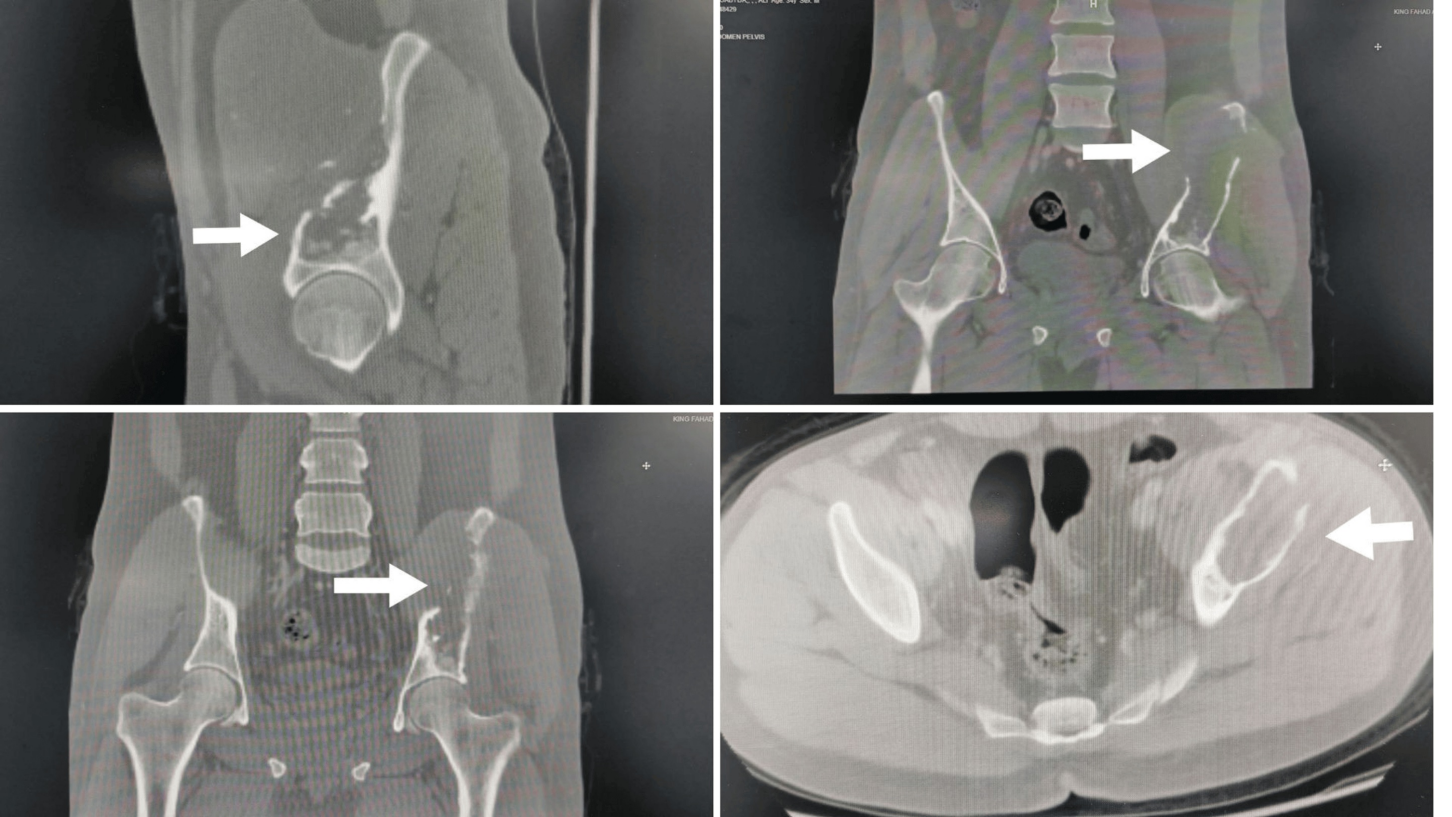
CT pelvis coronal, sagittal, and axial (bone window) demonstrate left iliac bone lytic lesion with evident of cortical breaching (arrows) CT, computed tomography
